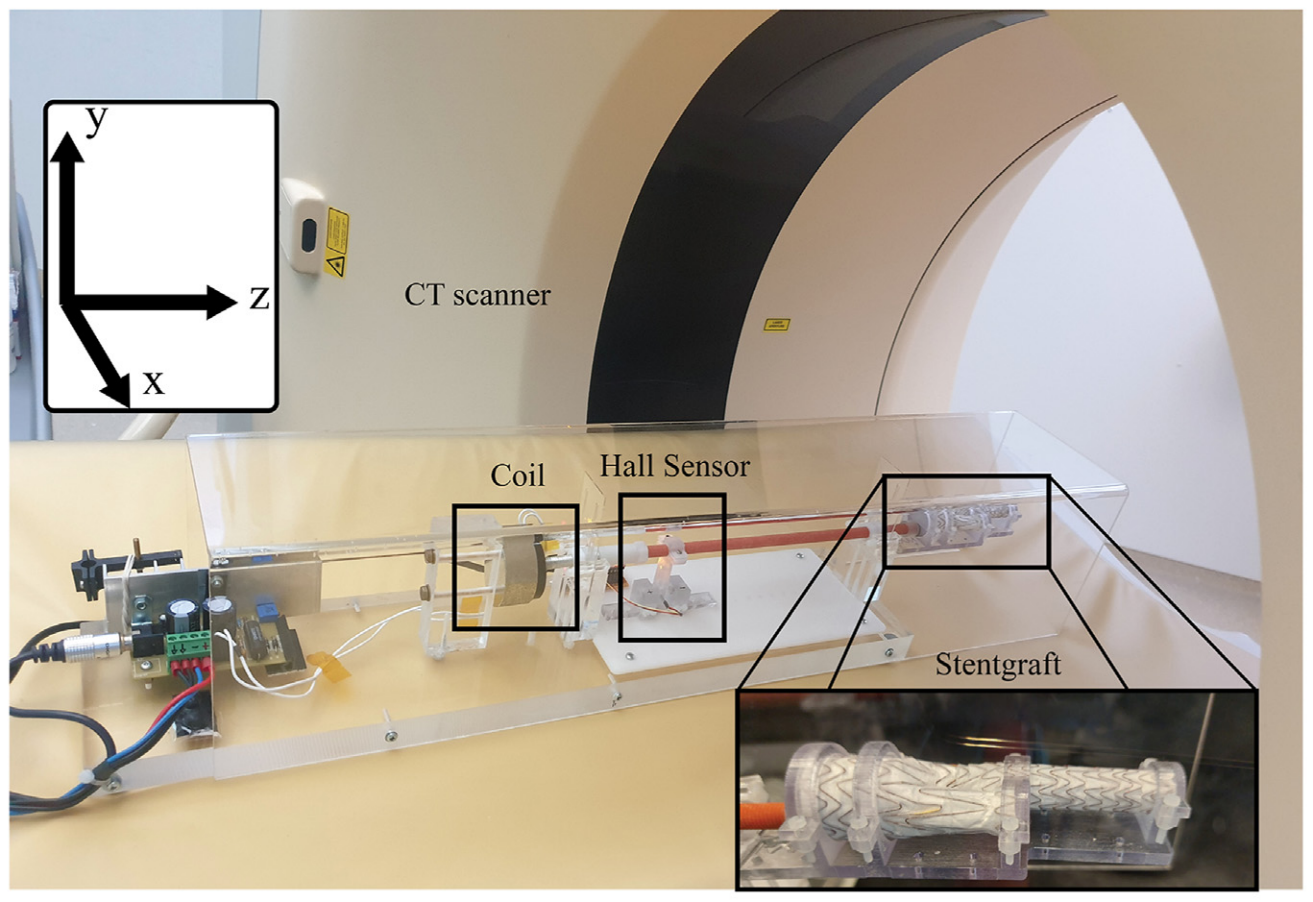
The experimental setup in a computed tomography (CT) scanner: The stent graft is moving in the z-direction (clinically the craniocaudal direction) of the scanner while attached to the red lever. This lever is stimulated by the triggered coil of the linear actuator. The hall sensor detects the true motion of system which can be interpreted using dedicated software.
